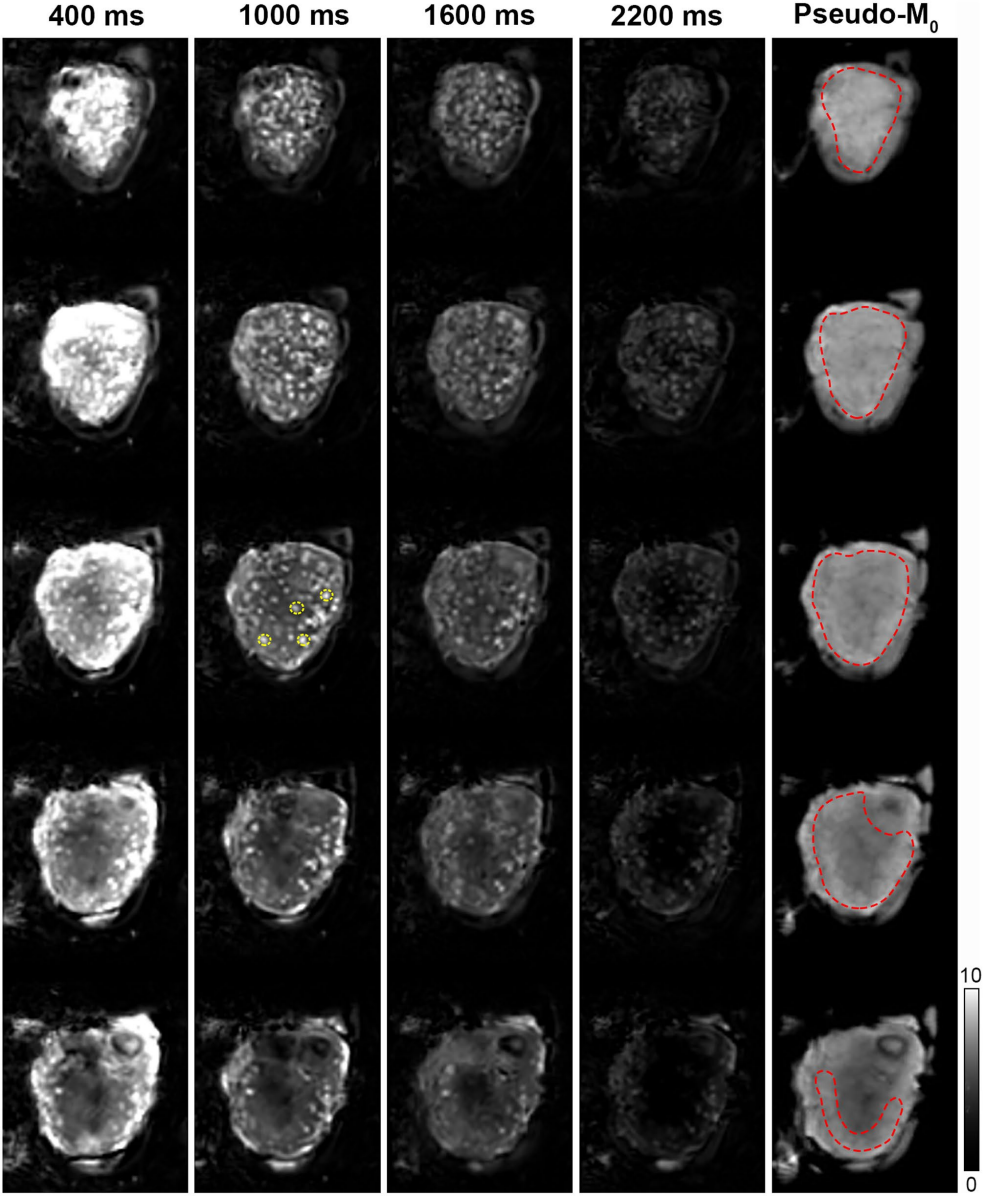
Variation of PLD. Placental perfusion images obtained with single VS‐ASL MRI using four different PLDs (400, 1000, 1600, and 2200 ms) and the corresponding pseudo‐M_0_ images in one pregnant volunteer (Subject 4 in Table 1). Multiple slices (spatial resolution: 4 × 4 × 4 mm^3^) with maternal coronal orientation were acquired in anterior to posterior order (top to bottom in Figure). ROIs were drawn manually in the placenta on the pseudo‐M_0_ images (ROI_placenta_; red dashed lines) and around four hyperintense focal regions identified on the perfusion images (ROI_focal_; yellow dashed lines on third slice of PLD 1000 ms). Perfusion images are shown with equal LUT scaling. The grayscale bar indicates PWS [%]
